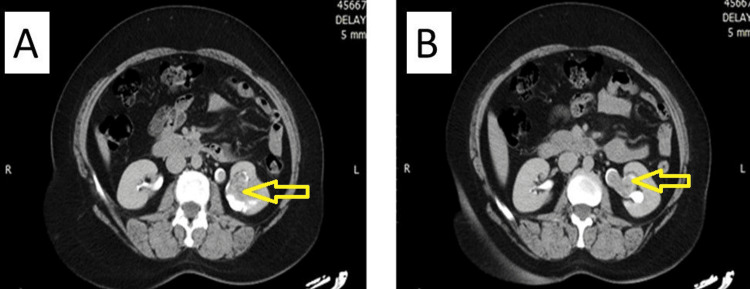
(A) A CT image showing a tumor arising from the lower pole of left kidney; (B) Extension of the tumor into the left renal pelvis CT: computed tomography
